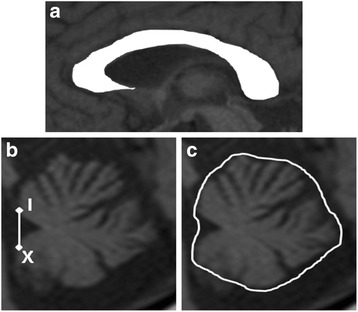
Segmentation of the corpus callosum and the cerebellum on MR images. The corpus callosum on a midsagittal T1-weighted image was extracted with the automated segmentation tool (a). The total area of the corpus callosum (mm^2^) was divided by the individual cranial AP diameter to correct for the individual head size differences as an area index (Adx). The cerebellum was defined as the area lateral to the line connecting the anterior lobe of the cerebellum (I segment) and cerebellar flocculus (X segment) (b). On the central slice, the fourth ventricle and surrounding cerebellar tissue were selected, and the cerebellum was automatically extracted using the imaging analyzer (c)
